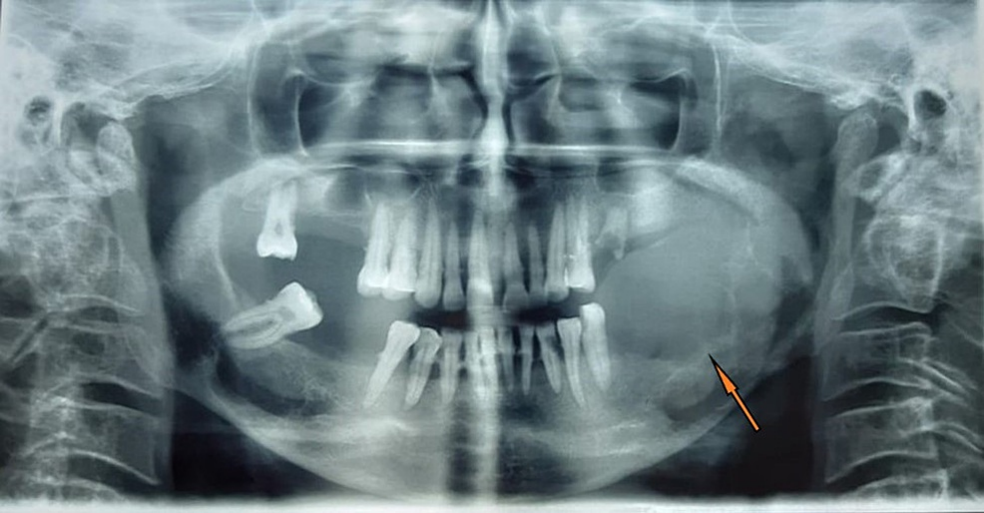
Orthopantomogram showing a well-defined radiolucent lesion extending from the lower left first premolar to retromolar region.
